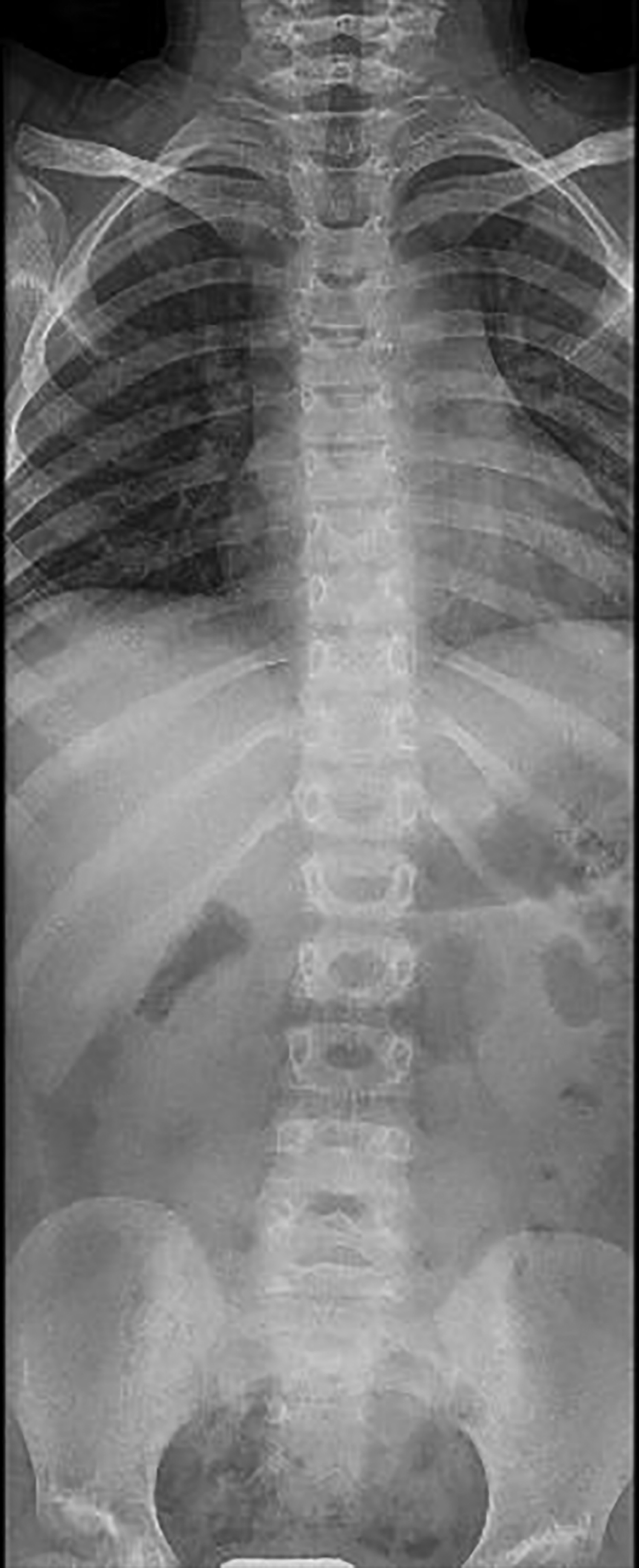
Radiography of the spine. X-ray of the spine shows on the coronal plane the presence of wide-radius convex left curvature of the thoracolumbar vertebrae.
